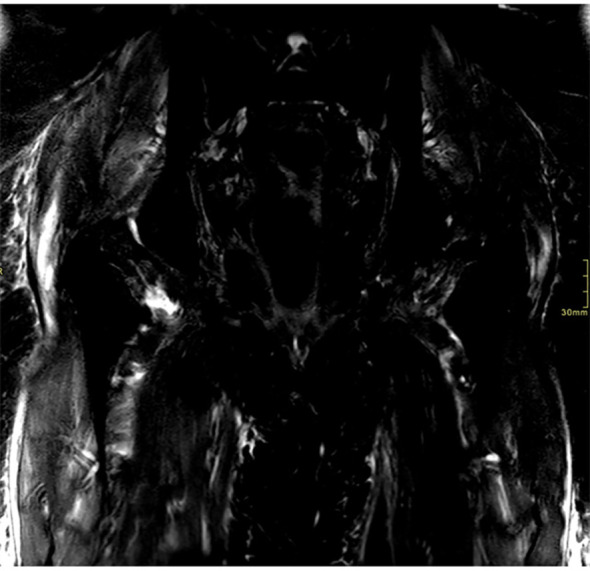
MRI of the pelvis and femurs with evidence of bilateral myositis - T2-weighted sequences STIR fat suppression.
